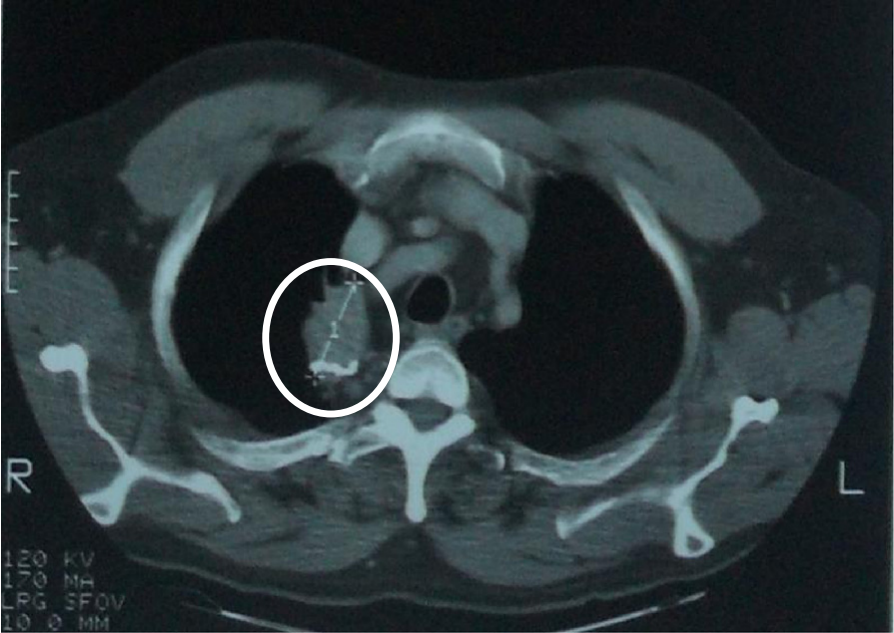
Thoracic CT Scan (1994): recurrent pulmonary lesion of alveolar echinococcosis after the right lobectomy performed in 1982.
